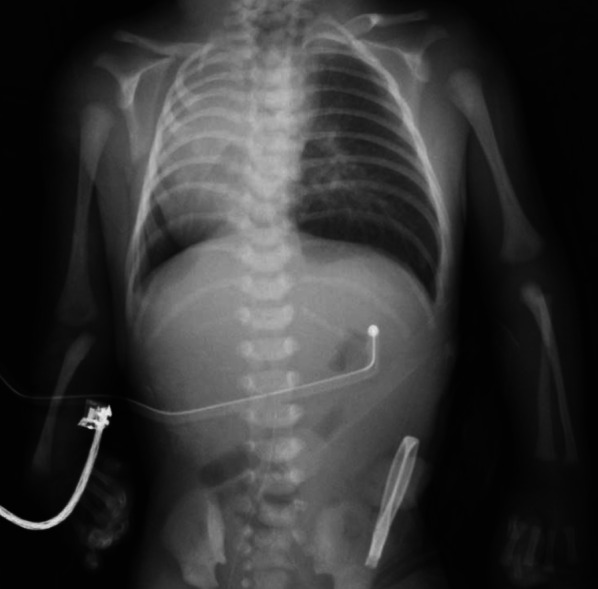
Chest X-ray findings after esophageal banding and gastrostomy was performed. The small right lung and mediastinal shift with dextrocardia and right radial hemimelia were detected
